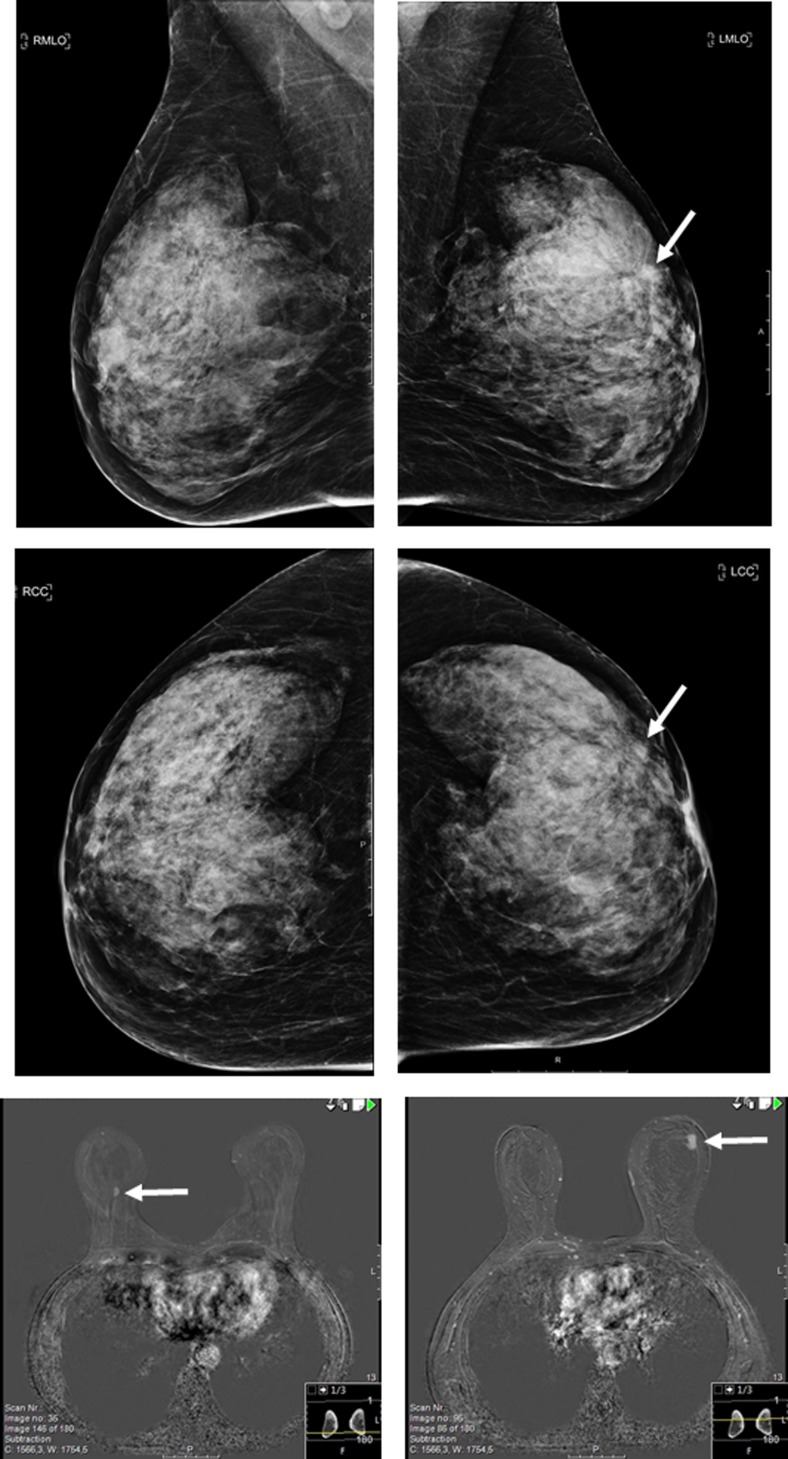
A 64-year-old woman was recalled for a BI-RADS 4 mass in the left breast. Percutaneous core biopsy showed invasive ductal carcinoma and MRI was performed to determine the extent of the disease. MRI demonstrated a BIRADS 4 mass in the right breast, which was occult at subsequent target ultrasound. MRI guided vacuum assisted biopsy revealed invasive lobular carcinoma. Breast conserving surgery yielded and invasive ductal carcinoma of 8 mm in the left breast (sentinel node negative, B&R II, ER+, PR+, Her2-) and an invasive lobular carcinoma of 6 mm in the right breast (sentinel node negative, B&R I, ER+, PR-, Her2-)
